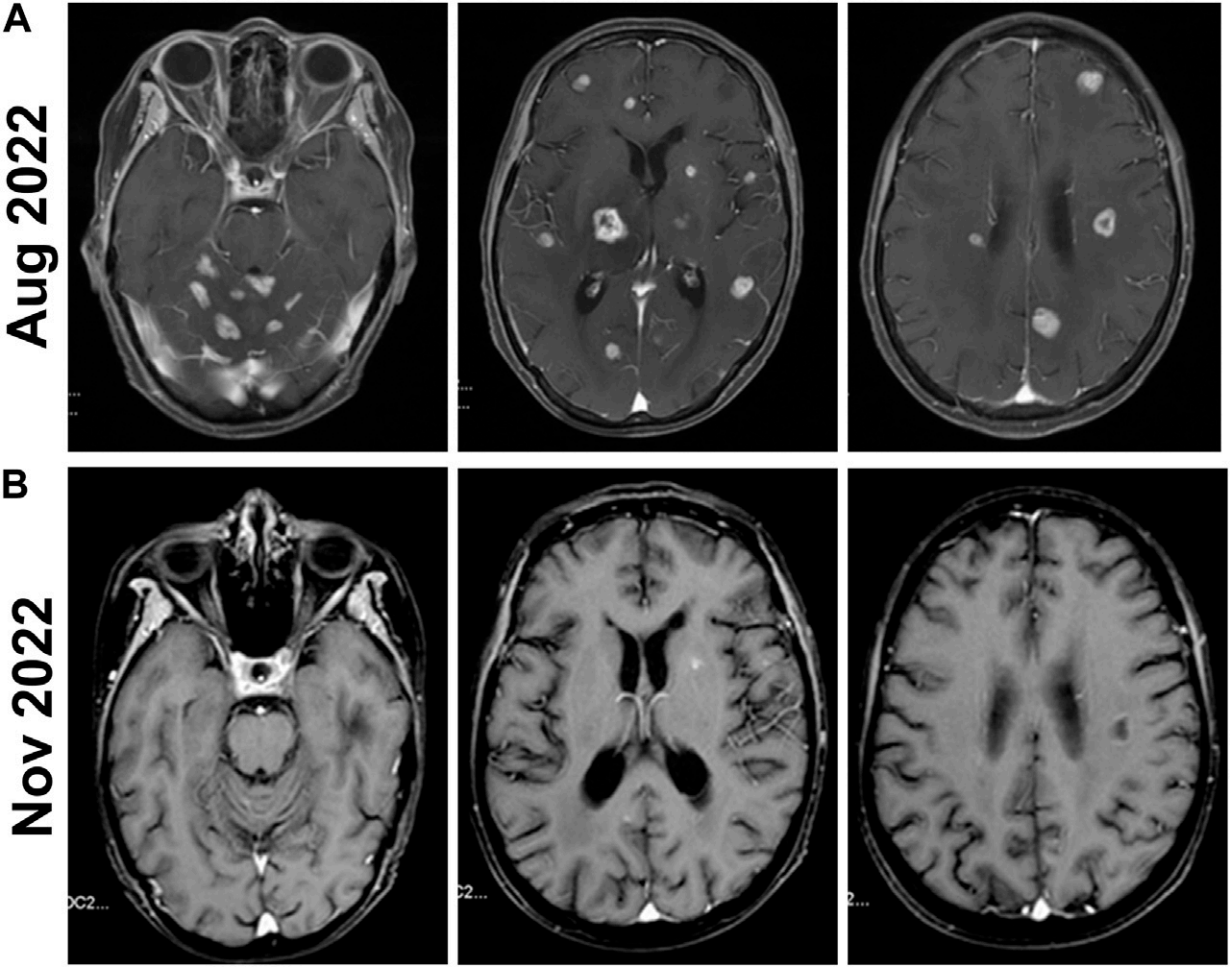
Brain MRIs of the patient before and after the Anlotinib treatment. (A) Before the Anlotinib treatment, the enhanced MRI of the head in August 2022 shows that the size and number of multiple brain parenchyma metastatic tumors have increased compared to previous scans. (B) The follow-up enhanced MRI of the head in November 2022 shows a significant reduction in the number and size of metastatic tumor lesions compared to previous scans.
